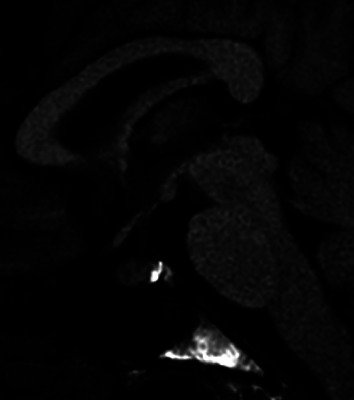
Pituitary contrast-enhanced MRI revealed heterogeneous enhancement of the pituitary gland.
